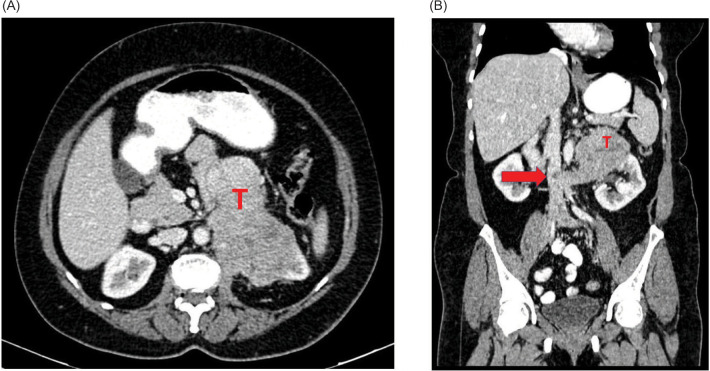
Computed tomography images showing the heterogeneously enhancing left renal mass involving the left renal vein and IVC and abutting the aorta. (A) Cross-sectional image and (B) Sagittal image. (T – Tumor), arrow pointing to the inferior vena cava (IVC) thrombus.
